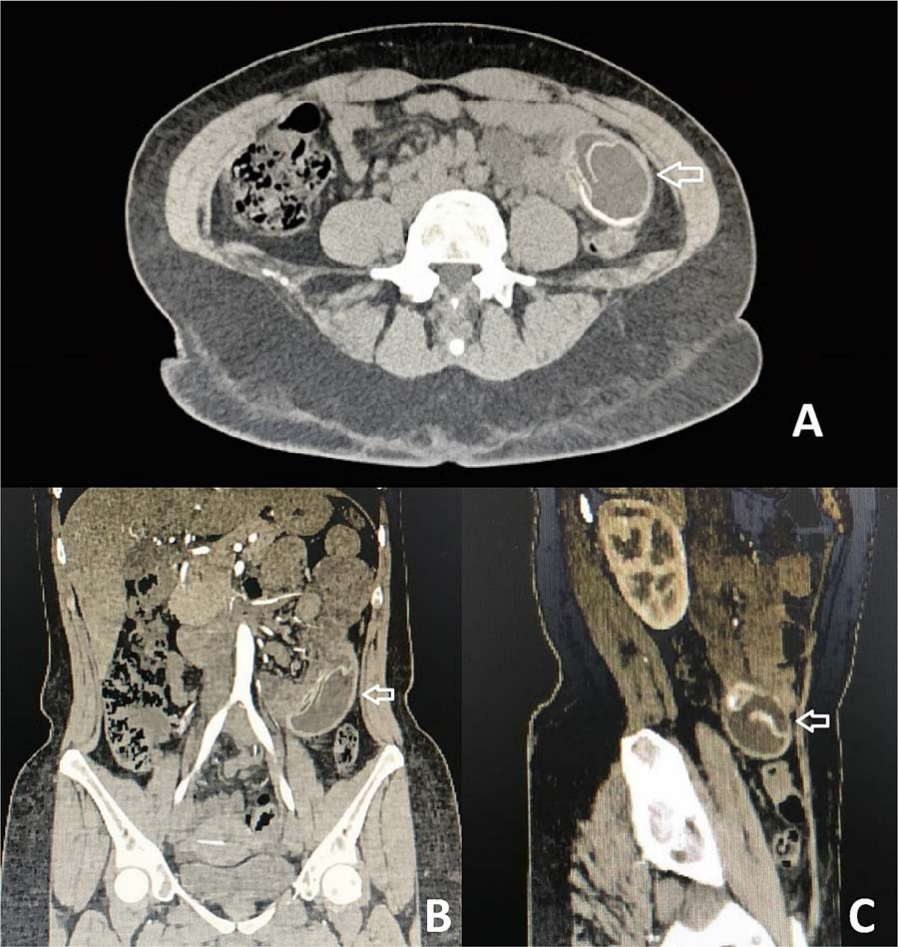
CT with evidence of a foreign body compatible with a migrated gastric balloon (indicated by the arrow). (A) Axial plane. (B) Coronal plane. (C) Sagittal plane.
